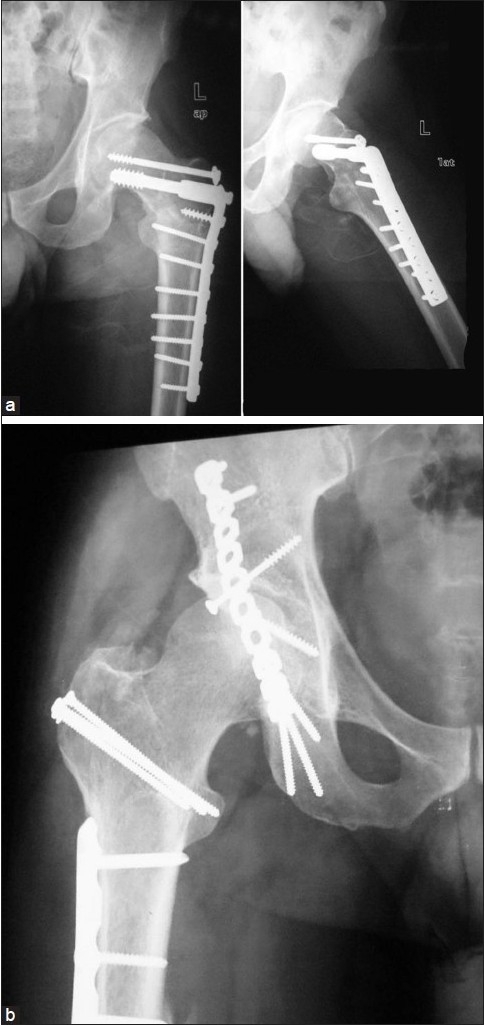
(a) Radiograph of the left hip with the thigh (an anteroposterior view) at 28 months showing a good union of both the fractures and no evidence of avascular necrosis. (b) Radiographs of the right hip with the thigh (an anteroposterior view) showing a good union at trochanteric osteotomy and acetabular fracture with no evidence of avascular necrosis
